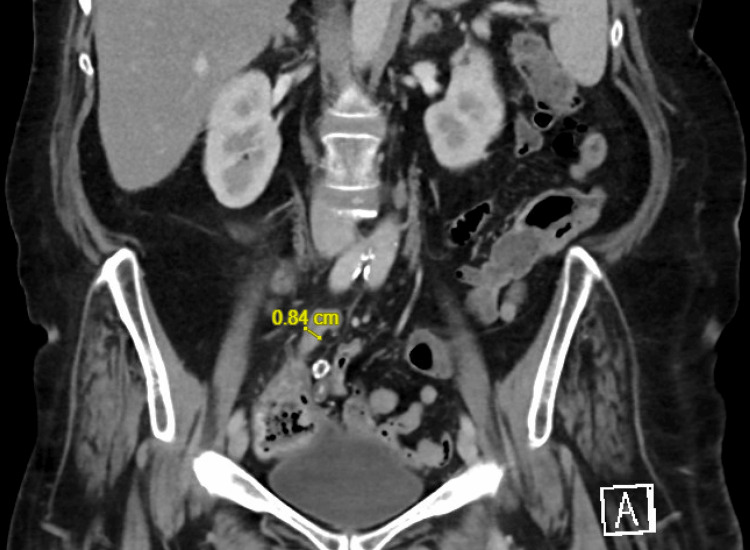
A CT scan showing acute appendicitis with the appendix diameter measuring 0.84 cm and surrounding mild inflammatory changes at the tip
